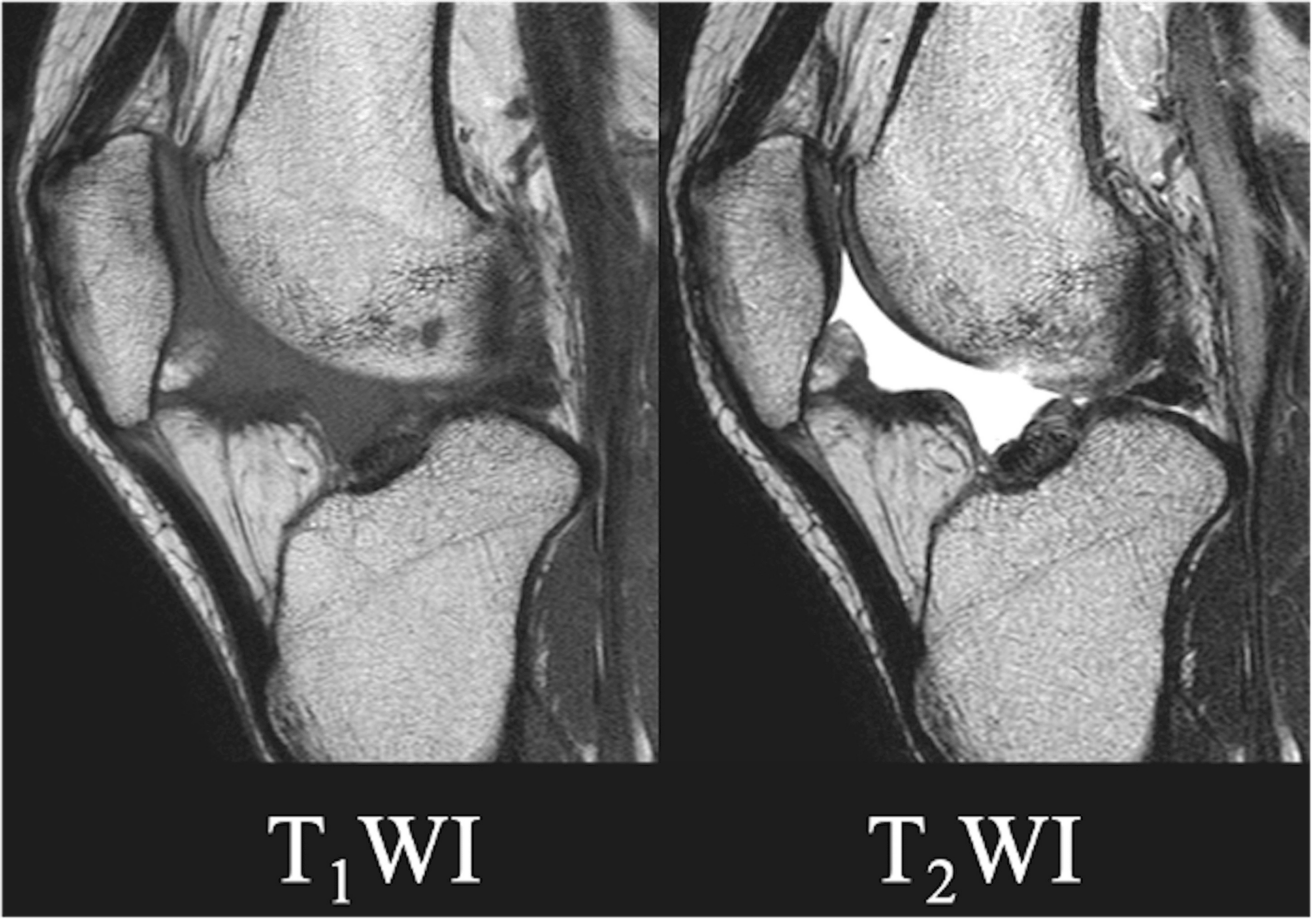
Magnetic resonance images of the knee (six months postoperatively) The ganglionic cyst was no longer visible.
